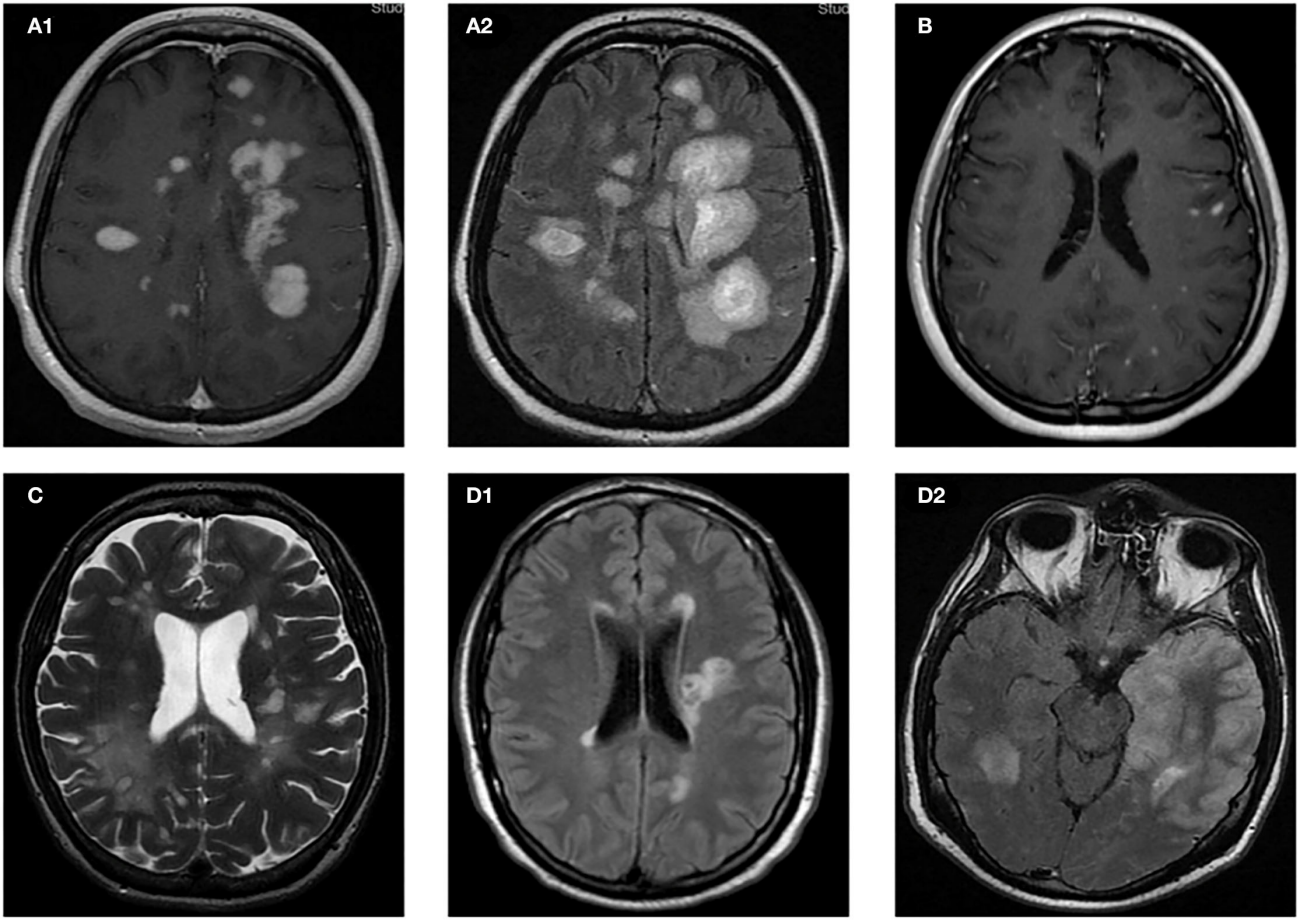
Brain MRI of the cases. (A) Case 1: axial T1 post-gadolinium sequence (A1) and FLAIR sequence (A2). (B) Case 2: axial T1 post-gadolinium sequence. (C) Case 3: axial T2 sequence. (D) Case 4: axial FLAIR sequence on day −1 (D1) and on day +21 (D2) with respect to vaccination.
